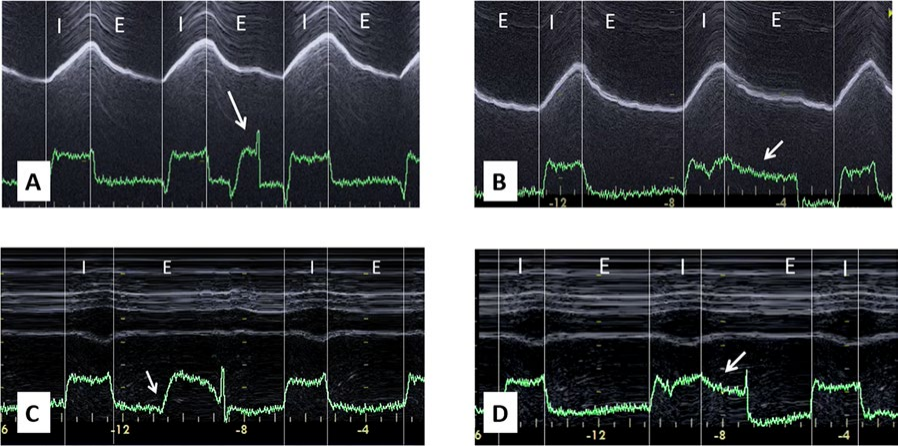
Diaphragmatic ultrasonography results
